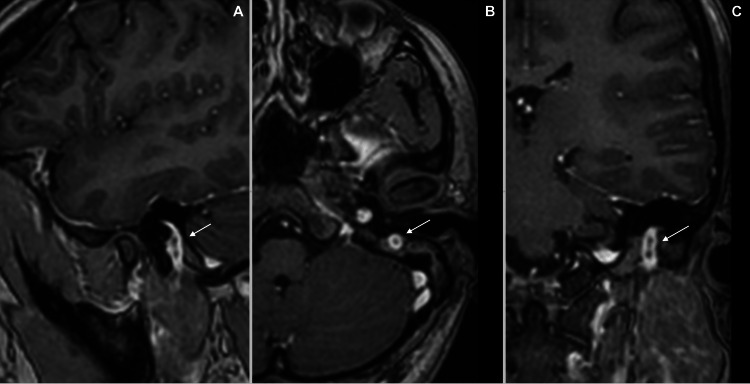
Gadolinium-enhanced T1-weighted image of MRI (internal acoustic meatus and brain). (A) Sagittal view, (B) axial view, and (C) coronal view. Arrow shows an enhancing left mastoid segment of the facial nerve causing the widening of the stylomastoid foramen. MRI: magnetic resonance imaging.
